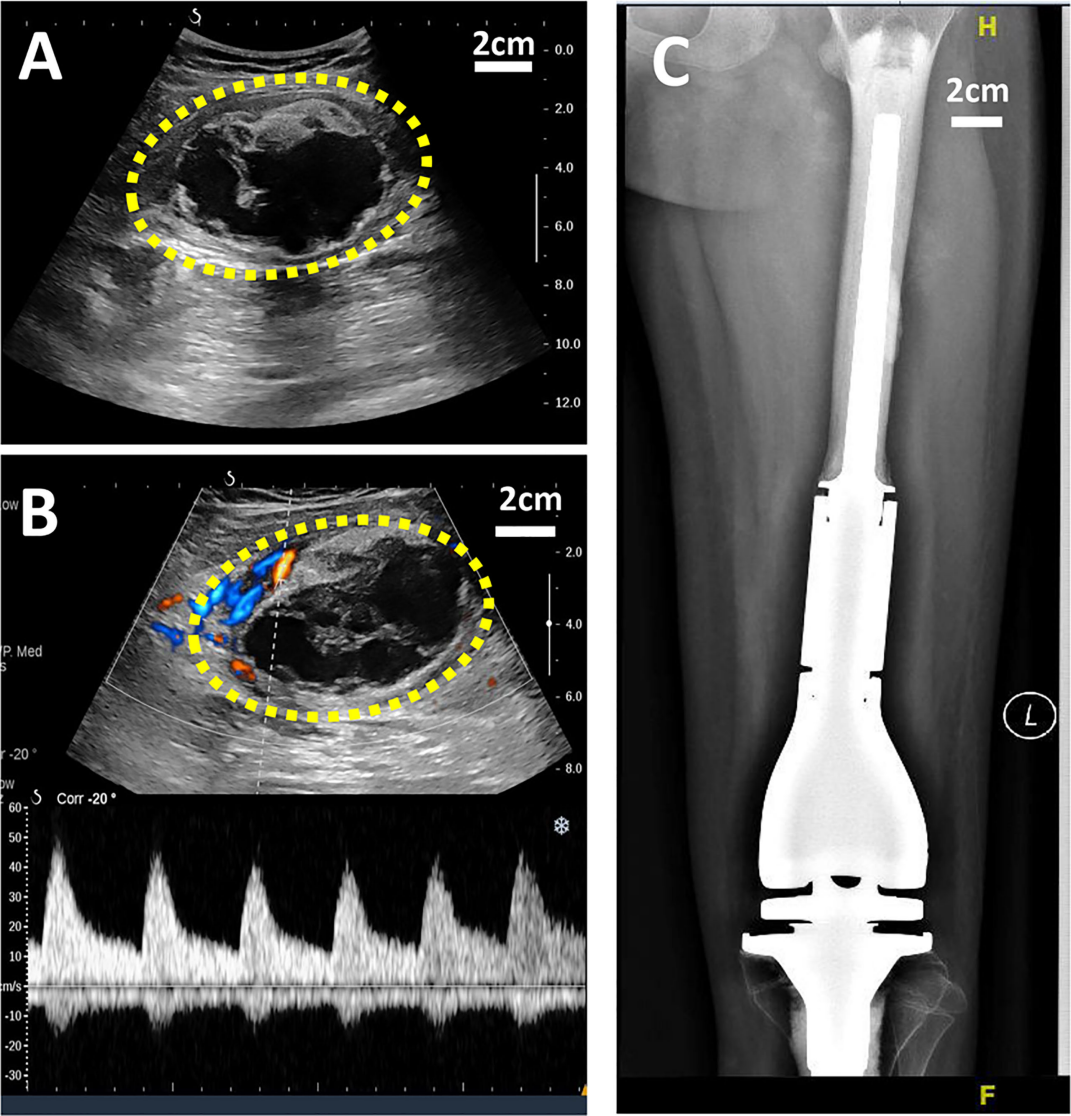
US and X-ray images of a 11-year-old girl with OS local recurrence after limb salvage with metal implants. It was easy to see the lesion in US but not in X-ray image. (A) A heterogeneous echogenic mass in the longitudinal plan of US image. (B) Color Doppler and power Doppler US image of the mass. (C) No obvious lesion was found I the X-ray image.
